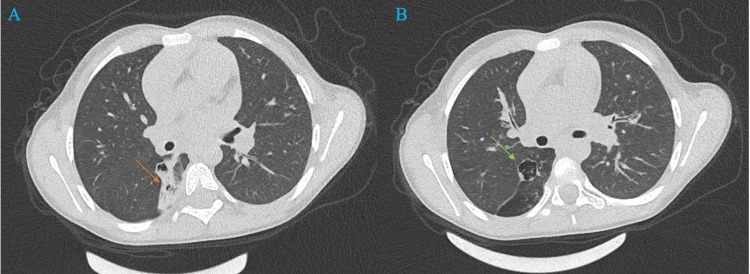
Axial chest CT (lung window) A: Right lower‑lobe parenchymal consolidation with air bronchograms (orange arrow); B: Thin‑walled, air‑filled cystic lesion in the posterior basal segment of the right lower lobe (green arrow).
